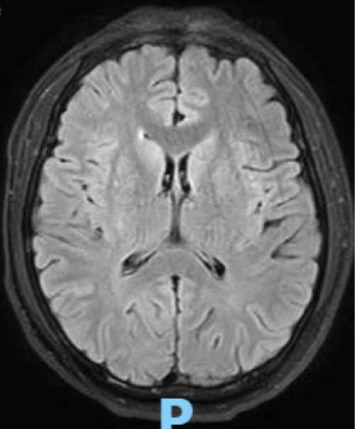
MRI finding shows normal parenchyma and ventricles, with no evidence of extra-axial fluid collection, mass lesion, or mass effect.
